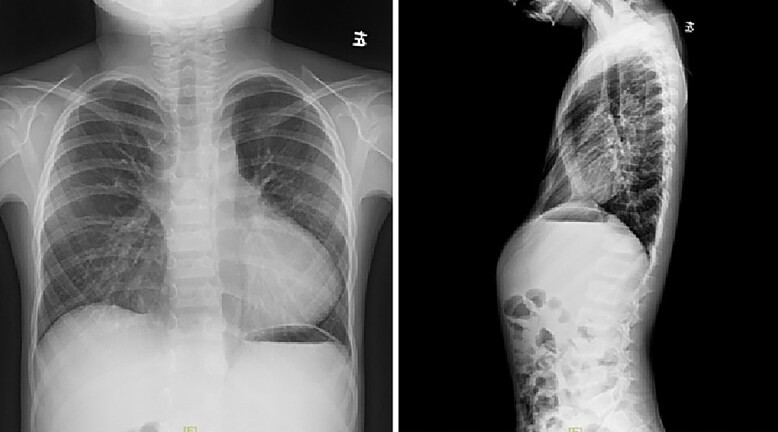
Chest X-ray examination before reoperation.
